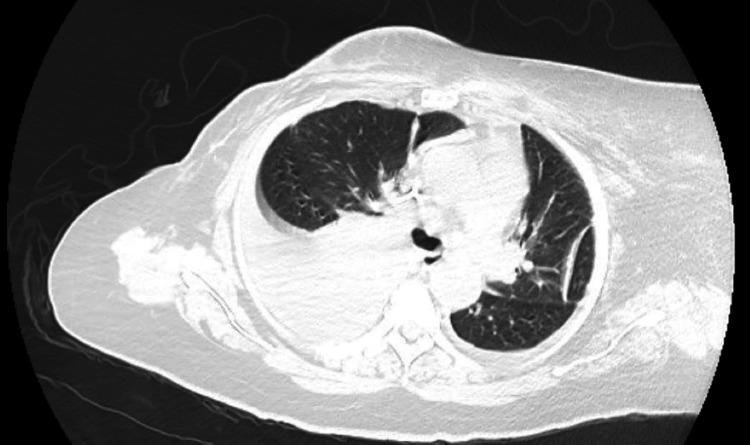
Chest CT of our patient demonstrating a left sided pleural effusion
